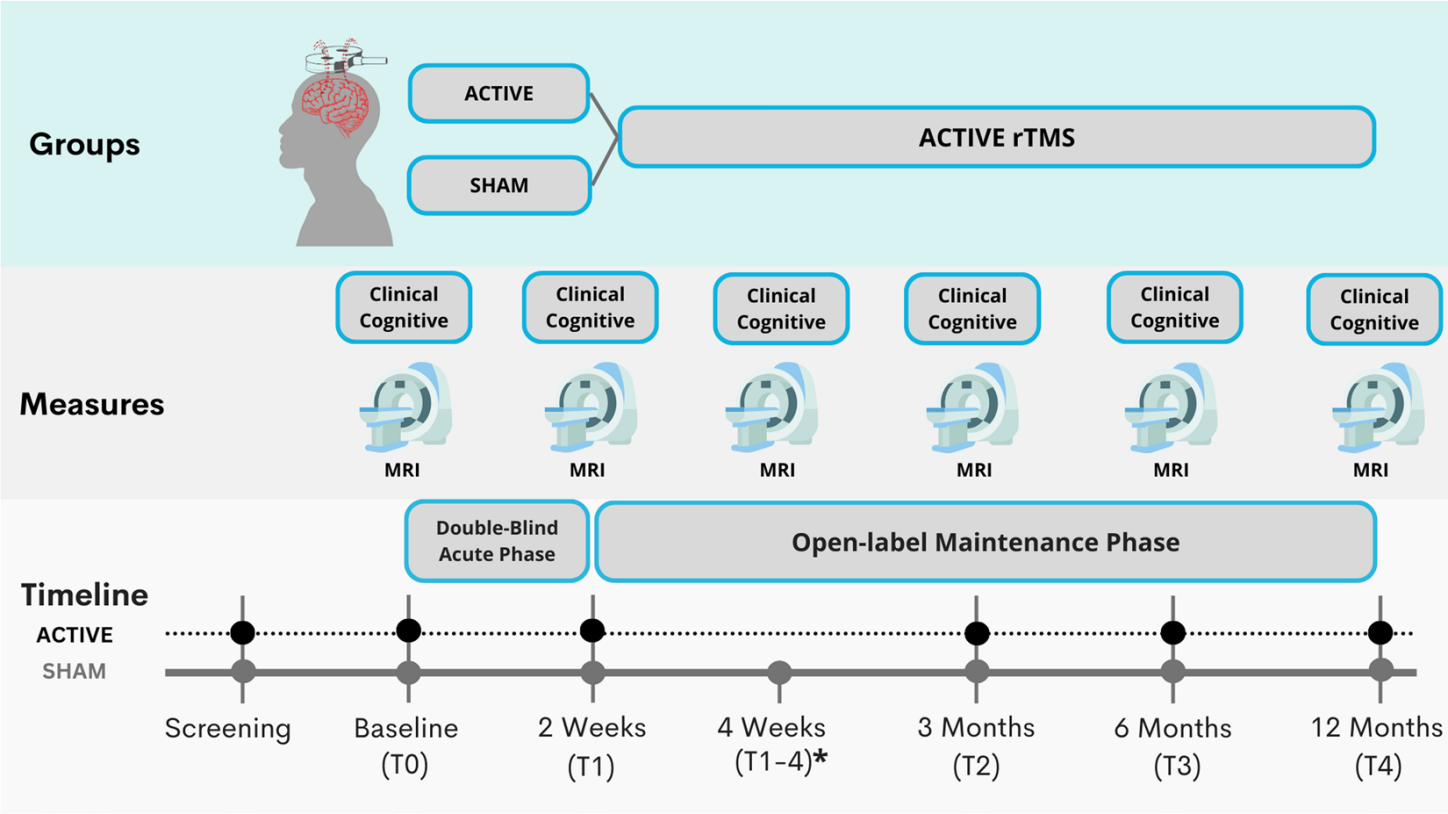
SUDMEX-TMS experimental design. Clinical and MRI data were collected at the time of baseline (T0), at two weeks (T1), three months (T2), six months (T3) and 12 months (T4); * Patients who received the sham treatment and then received active treatment (T1–4).
